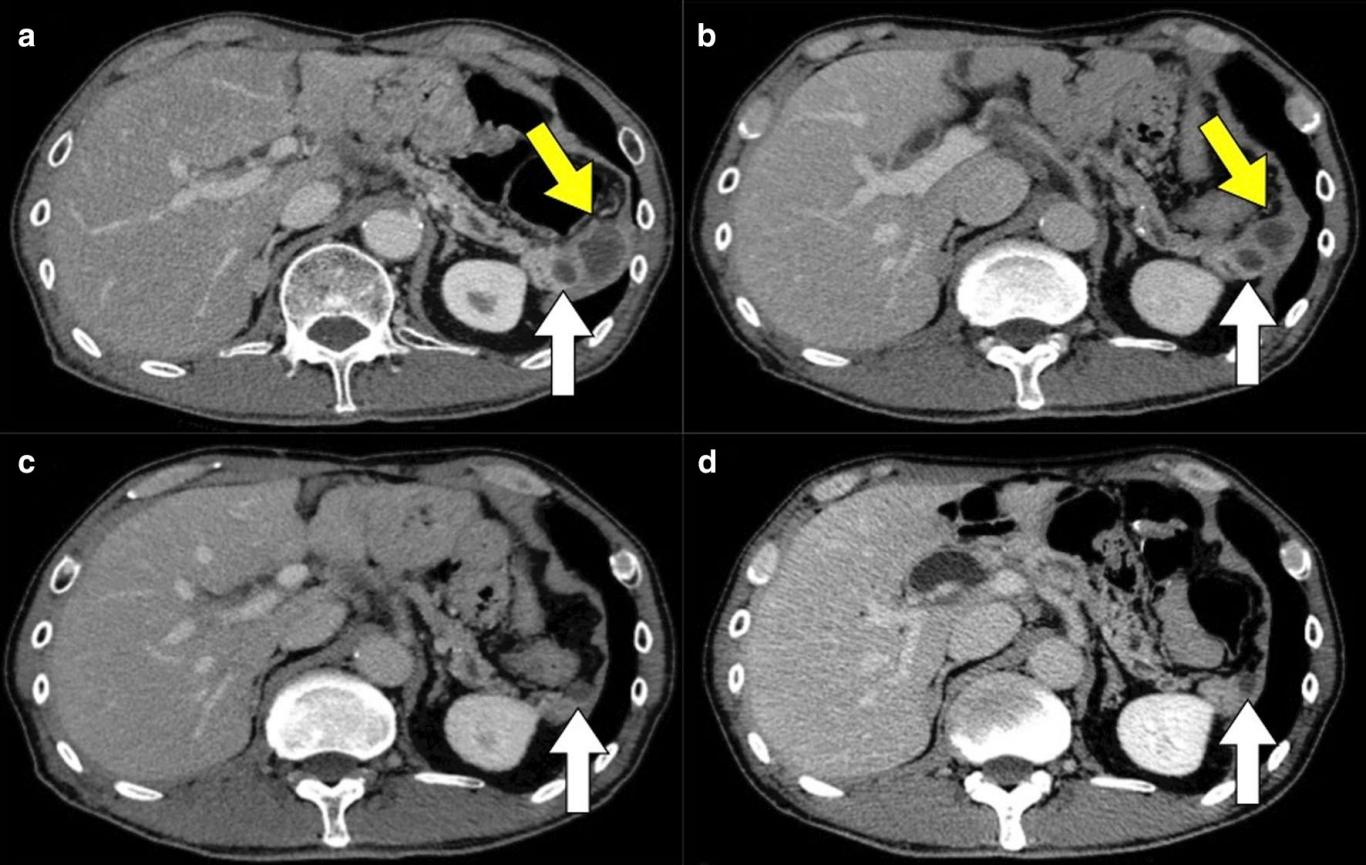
Contrast-enhanced computed tomography (CT) findings. CT showed a relatively clear, round, ring-enhanced tumor of 15 mm in diameter (white arrow) and an adjacent tumor of 35 mm in size (yellow arrow) (a). The larger tumor was enlarged compared with 1 month before (b) and had not been recognized 6 months previously (c). The smaller tumor had not changed from 3 years previously (d)
